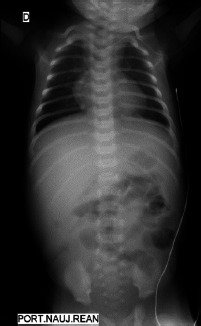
X-ray before operation showing no signs of perforation
